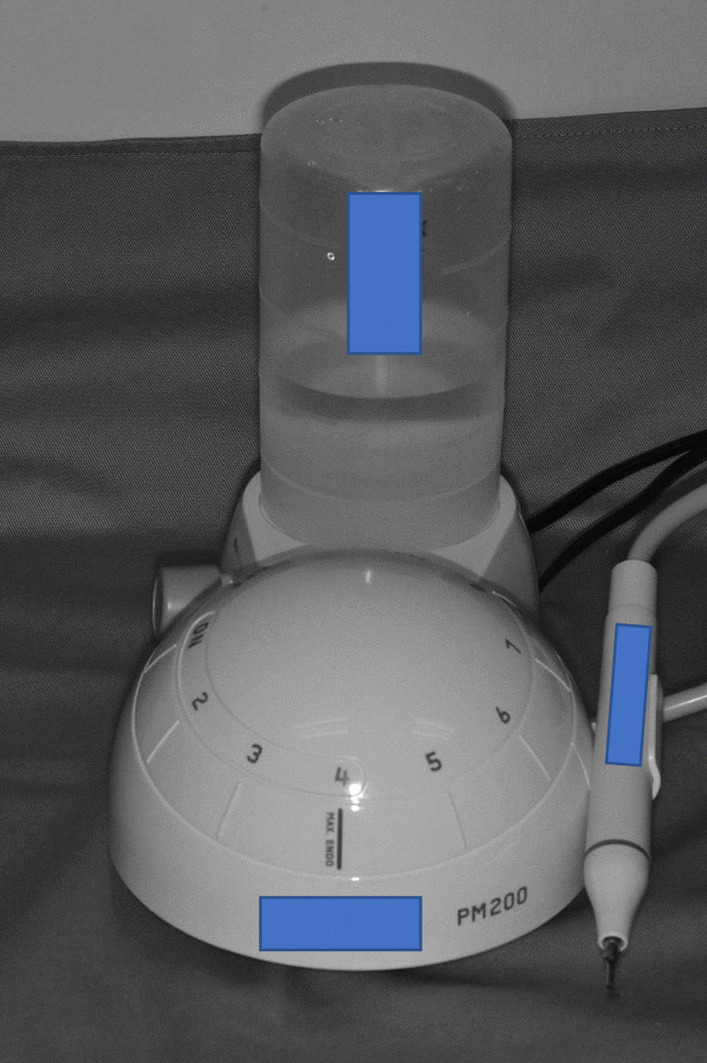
Portable ultrasonic scaler unit (PM200, EMS Switzerland)
